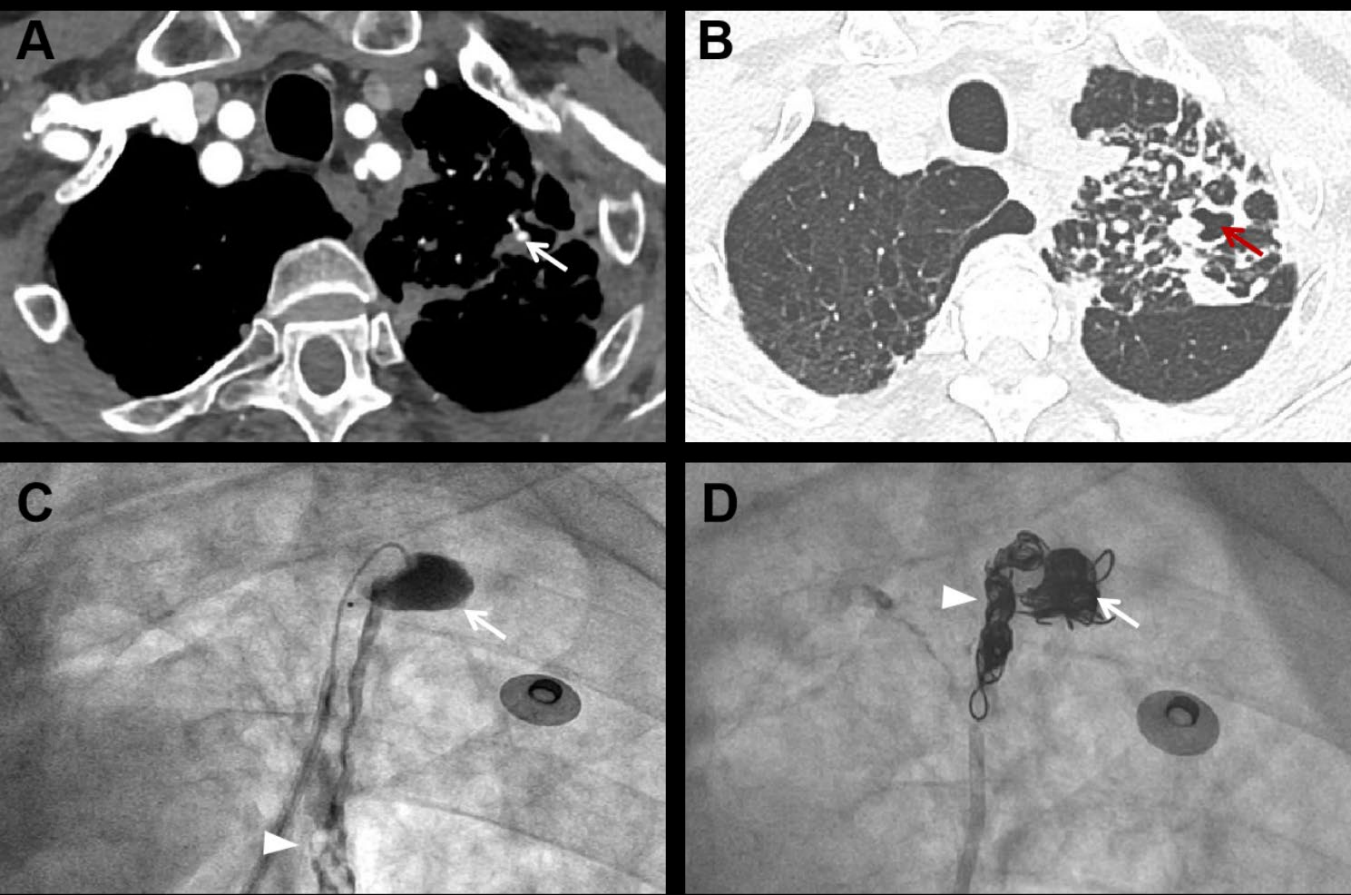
Representative images of ruptured pulmonary artery pseudoaneurysm. A 76-year-old male was hospitalized due to sudden massive hemoptysis. The patient had a history of tuberculosis and a positive sputum smear indicated the recurrence of tuberculosis. (A) Axial contrast-enhanced CT scan shows a pseudoaneurysm (arrow) distributed in the segmental pulmonary artery of the left upper lobe; (B) Axial contrast-enhanced CT scan shows that the PAP is adjacent to a cavitary lesion (red arrow); (C) Selective subsegmental pulmonary arteriography shows contrast agent extravasation from the PAP to the cavity (arrow) and trachea (arrowhead), confirming its rupture; (D) Final angiography shows occlusion of pseudoaneurysm (arrow) and the proximal of the feeding vessel (arrowhead) after coil embolization
